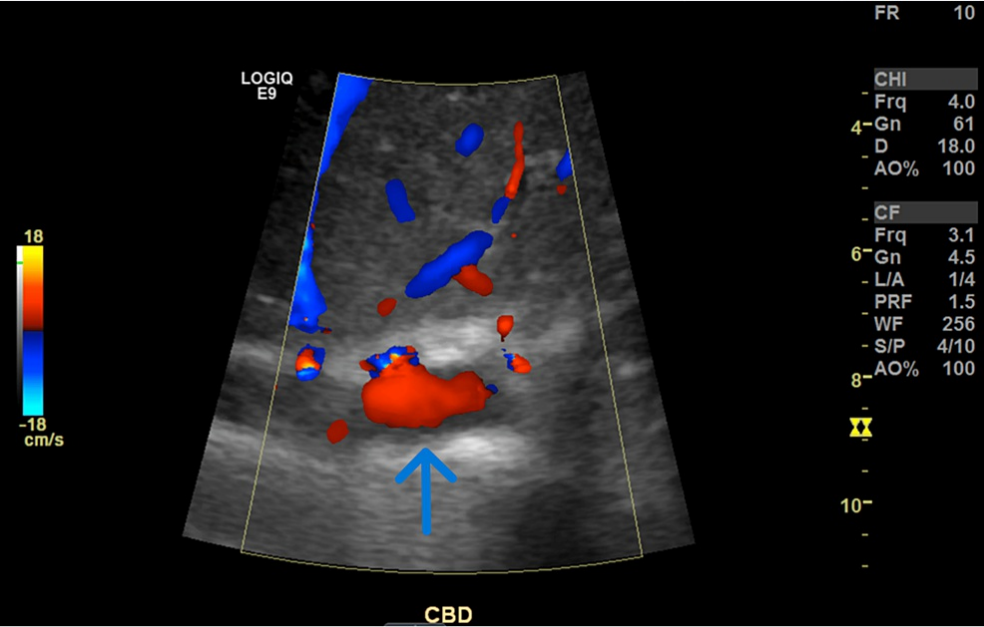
Abdominal ultrasound (US) revealing no intrahepatic ductal dilatation with a nondilated common bile duct (CBD) measuring 3 mm
